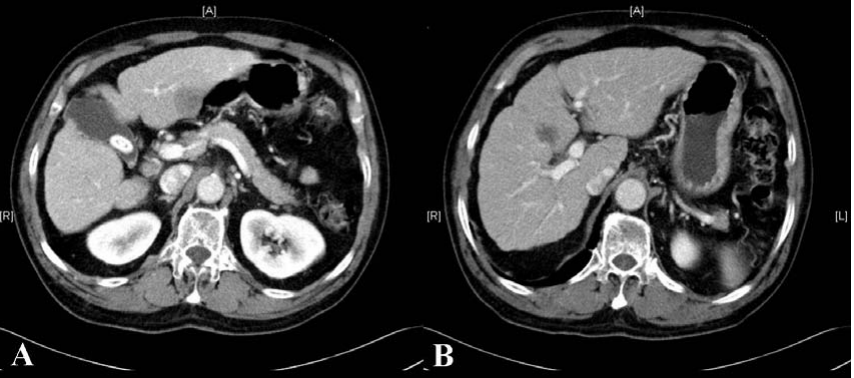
Abdominal computed tomography (CT) revealing two separate, low-density, 2-cm masses on segment II (A) and IV (B) of the liver.
